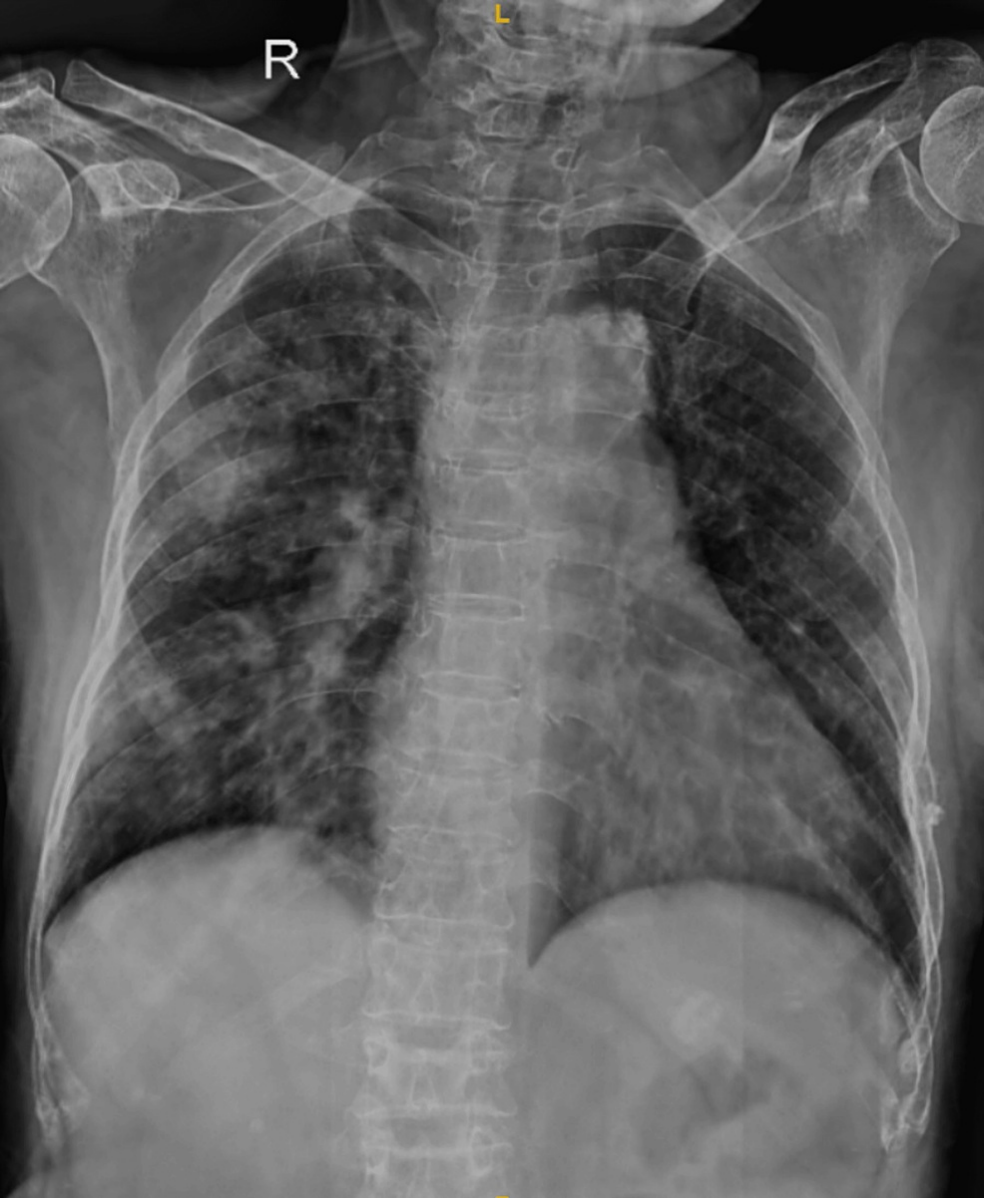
Chest X-ray showing bilateral bronchopneumonia
